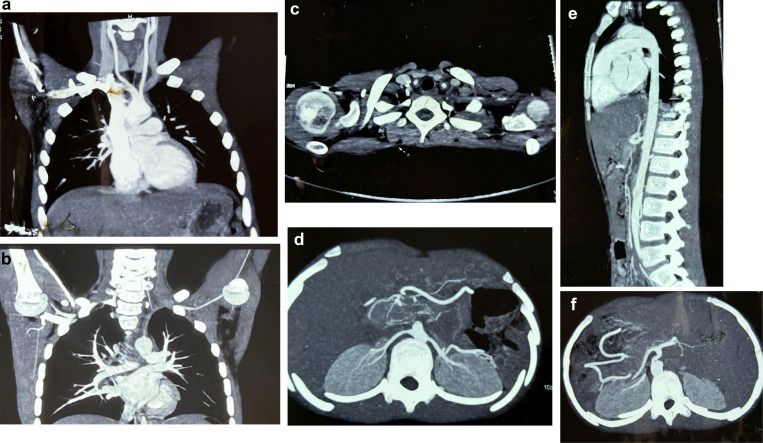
Panel of CT angiograms showing extent of vascular involvement in patient 4. (a–f) CT angiography of thoracic and abdominal vessels shows long segmental occlusion/tight stenosis of the entire length of the left subclavian artery with associated mild circumferential mural thickening. Left vertebral and left axillary arteries show attenuated caliber. Mild circumferential mural thickening involving the right and left common carotid arteries from the origin to bifurcation (c). Aortic root, ascending aorta, arch of aorta, descending thoracic aorta, abdominal aorta and its branches, bilateral common iliac arteries, bilateral external iliac arteries, and bilateral internal iliac arteries are normal in caliber and opacification (d–f).
